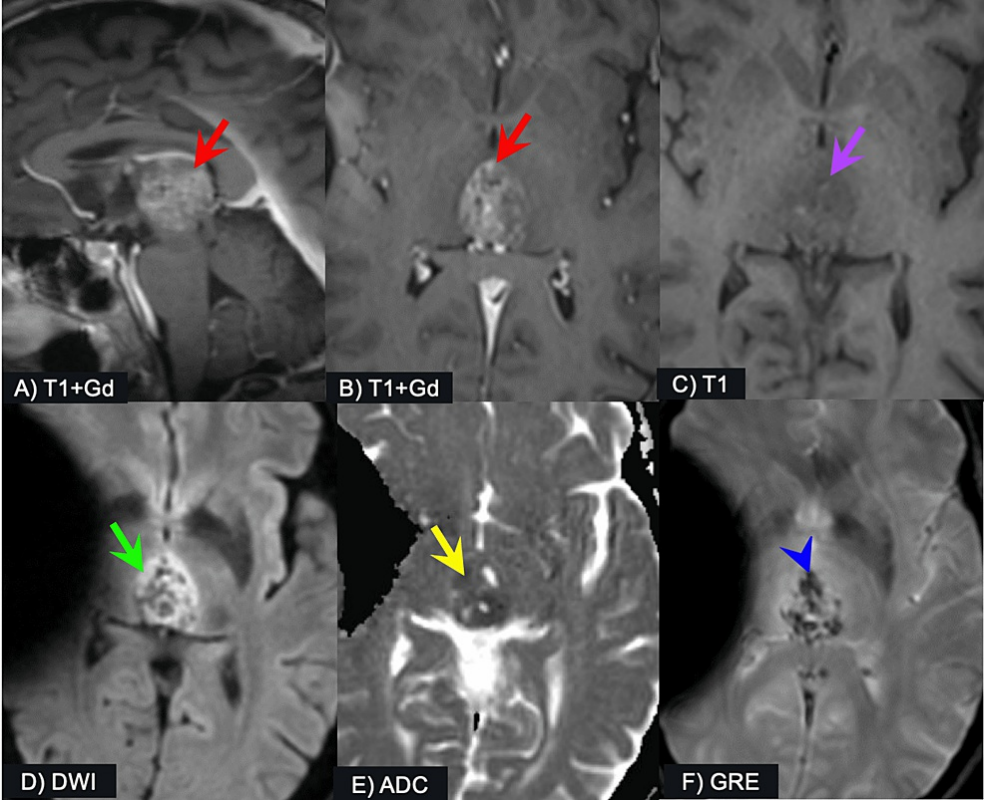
Images from MRI A heterogeneously enhancing and fairly well-circumscribed mass is seen centered at the pineal region (A, B: red arrows), which shows indistinct internal T1-hyperintense foci (C: purple arrow). Diffusion-weighted imaging (DWI) showed areas of restricted diffusion (D: green arrow) and low apparent diffusion coefficient (ADC) values indicating high cellularity (E: yellow arrow). Gradient echo sequences (GRE) revealed internal areas of hemorrhage and calcifications (F: blue arrowhead). Note: artifact present on axial images due to ventriculoperitoneal shunt. MRI - magnetic resonance imaging, Gd - gadolinium contrast
